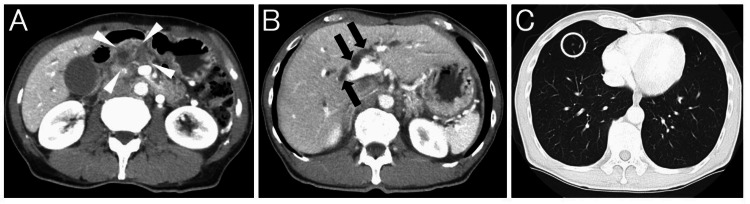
Contrast-enhanced computed tomography scan images at the time of the visit to our hospital. A: A tumor is found in the pancreatic head (white arrowhead). B: Bile duct upstream of the tumor is dilated (black arrow). C: A tumor is also found in the lung (circled).
